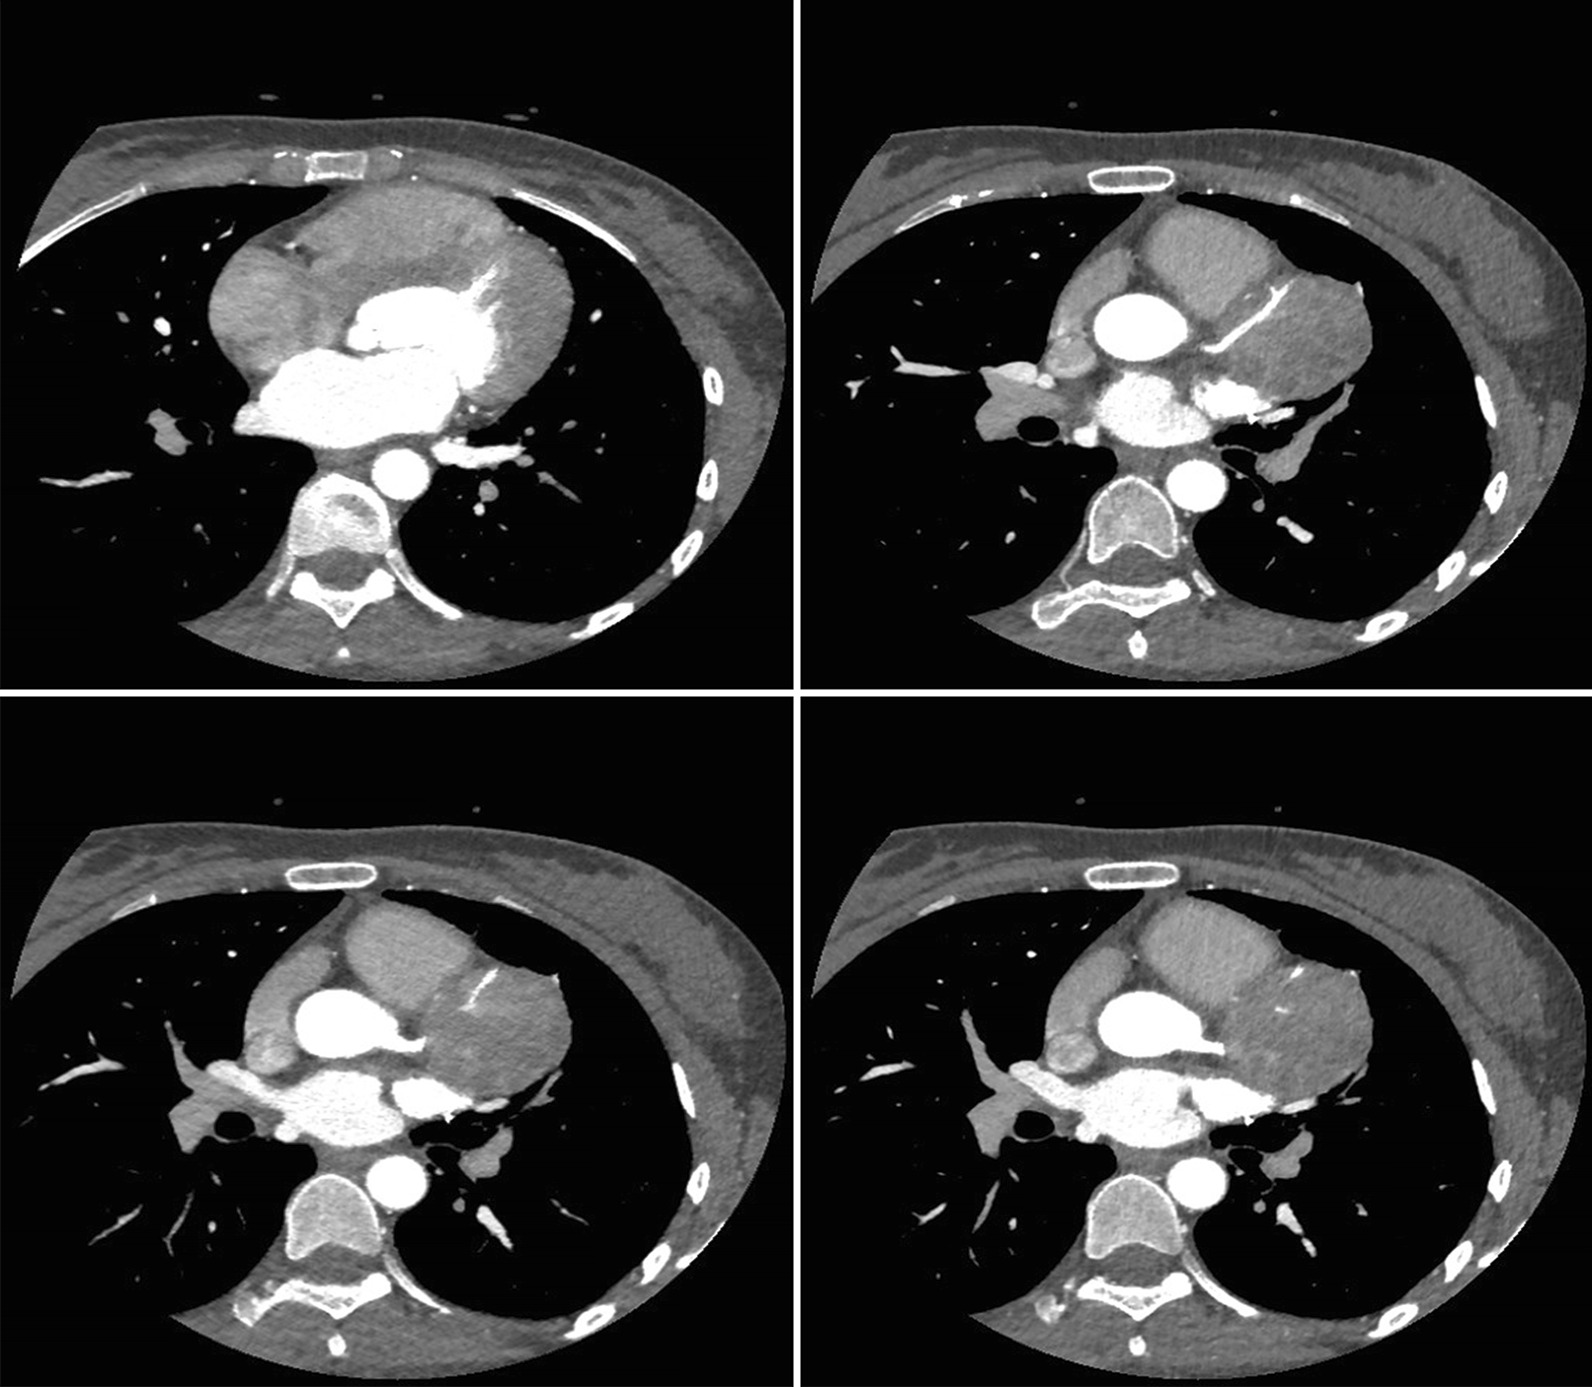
The imaging characteristics of PNET on coronary CTA at recurrence. 64-slice coronary CTA showed a solid and cystic lesion in the pericardial cavity, which circled the coronary artery
